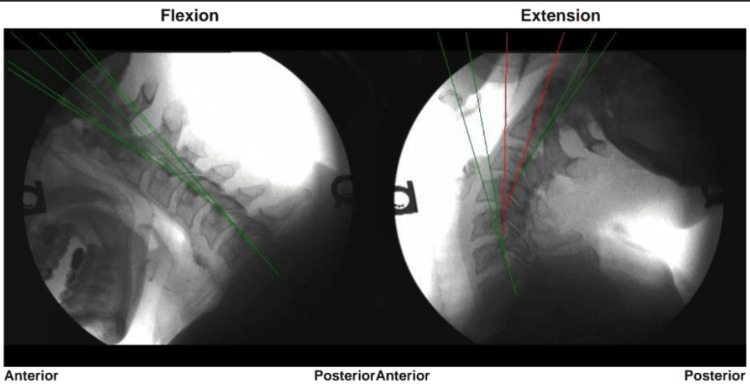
DMX image(s) - 2 Flexion and extension positioning of the cervical spine, with red lines showing abnormal alignment and green lines demonstrating normal alignment DMX: digital motion X-ray
